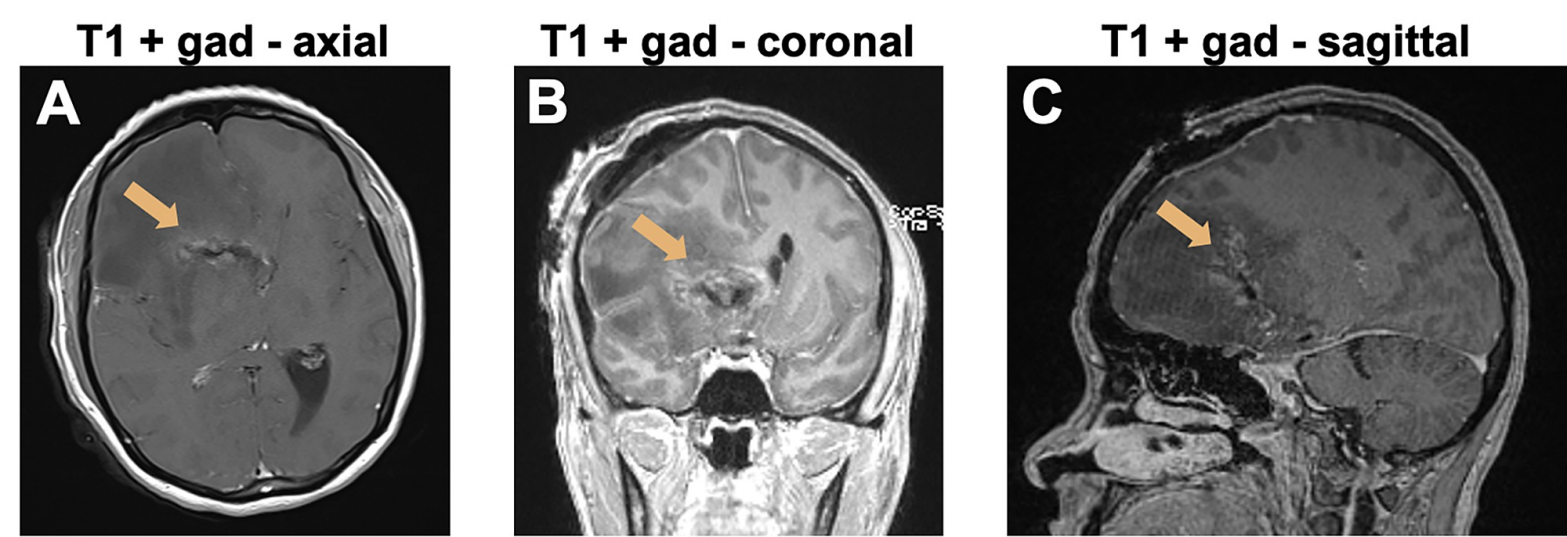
Postoperative MRI A, B, C: T1-weighted MRI with gadolinium enhancement on postoperative day 1 shows gross total resection of the enhancing mass (arrows).
